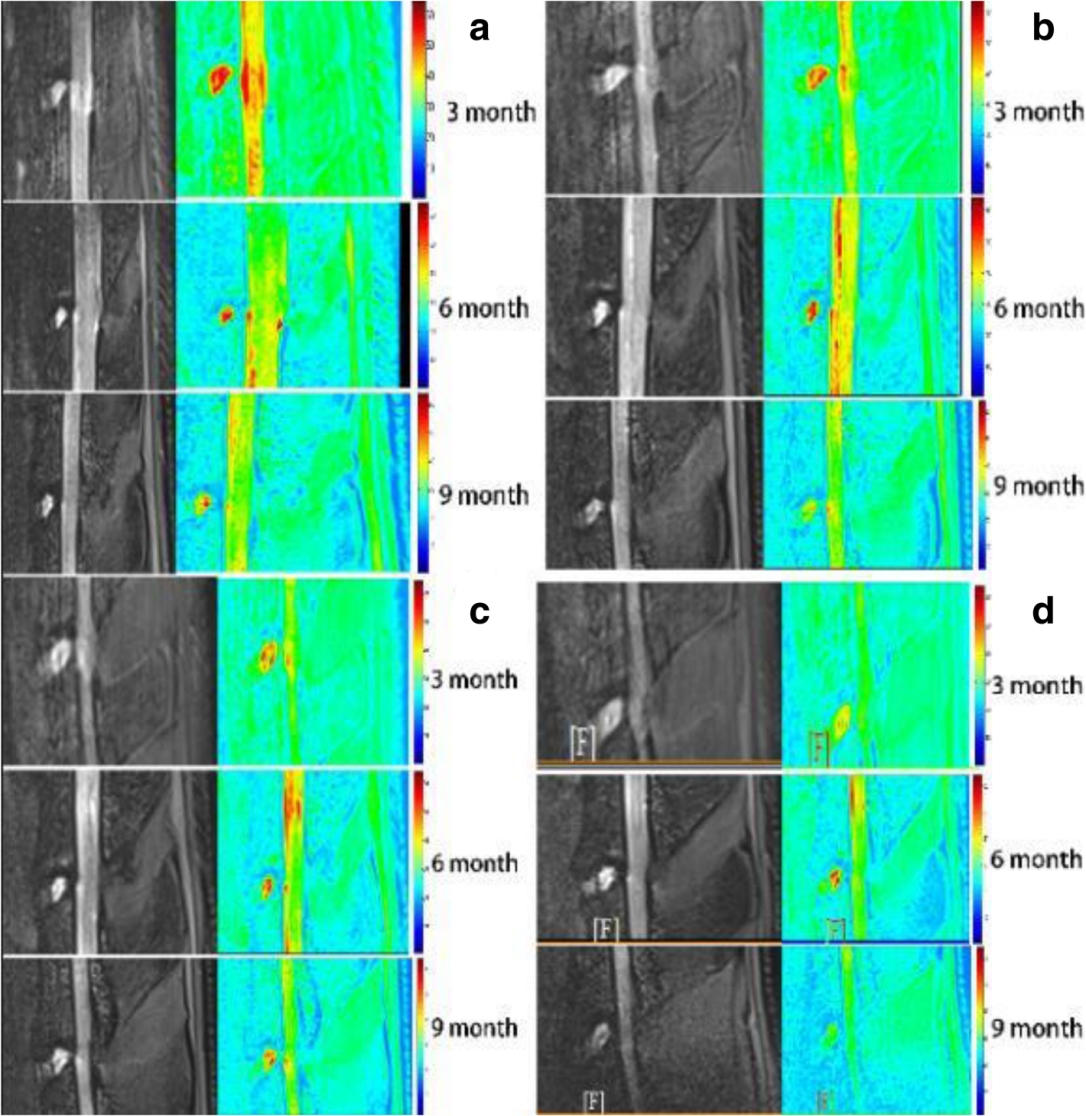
Sagittal T2-weighted images (gray images) and corresponding T2 maps (colored images) of discs with different levels. Relaxation times (ms) are displayed on the right of each T2 map image. a Intervertebral disc in L3–L4. b Intervertebral disc in L4–L5. c Intervertebral disc in L5–L6. d Intervertebral disc in L6–S1
